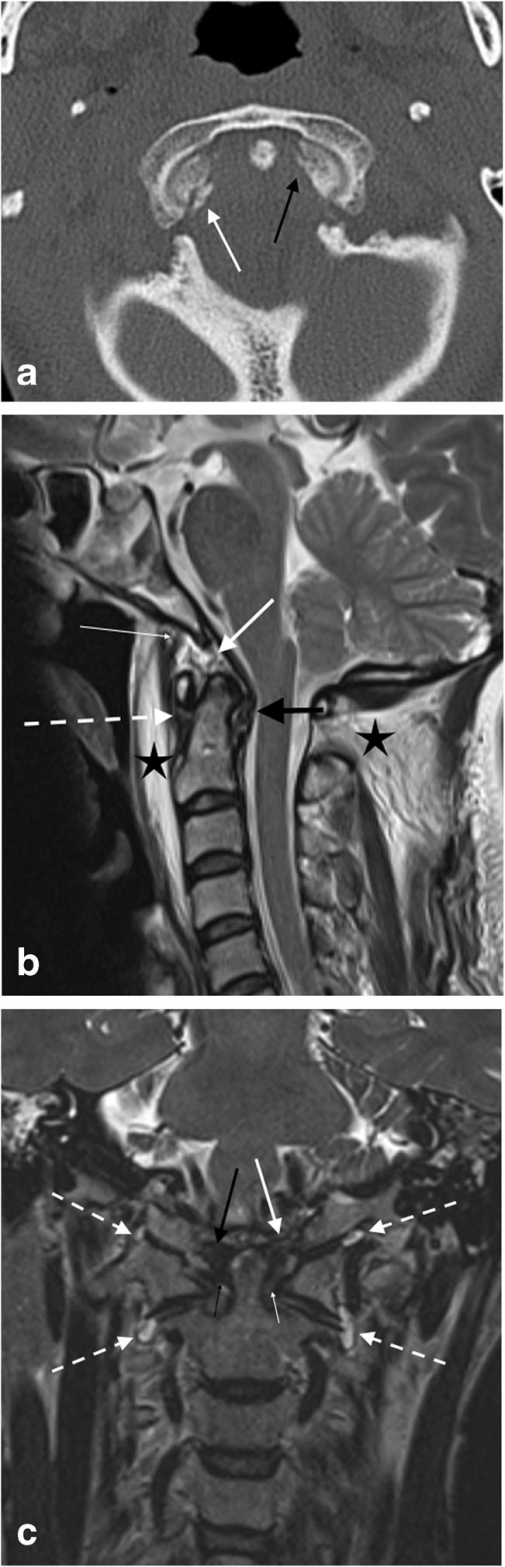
Multi-focal craniocervical junction osseo-ligamentous traumatic injury in a 51-year-old male driver of a vehicle which collided with a wall at high speed: a: axial CT image through the atlanto-occipital level demonstrates type —III left occipital condyle fracture (*black arrow*) and type I right occipital condyle fracture (*white arrow*); b: sagittal T2-weighted image through the craniocervical junction of the same patient—there is oedematous strain injury affecting the apical ligament (*bold white arrow*) with haemorrhagic fullness of the surrounding supra-odontoid space (apical cave; not annotated). There is strain injury of the transverse ligament with peri-ligamentous fluid surrounding the ligament (*black arrow*) as well as widening of the anterior atlanto-dental space (not annotated). There is partial tear of the basion attachment of the anterior atlanto-occipital membrane (*thin white arrow*) and traumatic disruption of the anterior atlanto-axial membrane (*severed white arrow*). Sizeable prevertebral haematoma and traumatic posterior paraspinal soft tissue oedema are also present (*black asterisks*); c: coronal image of T2-SPACE sequence through the craniocervical junction of the same patient demonstrates traumatic signal abnormality and partial tear of the left alar ligament (*long white arrow*) compared with the greater integrity of the right alar ligament (*long black arrow*), abnormal signal related to disruption of the left transverse ligament (*short thin white arrow*) compared to the integrity of the right side of the ligament (*short thin black arrow*). Traumatic effusions are evident affecting both the atlanto-axial joint capsules (Arnold’s ligaments) and the atlanto-occipital joint capsules bilaterally (*severed white arrows*). Bilateral traumatic otomastoid effusions are present (not annotated) related to lateral skull base fractures (not depicted)
